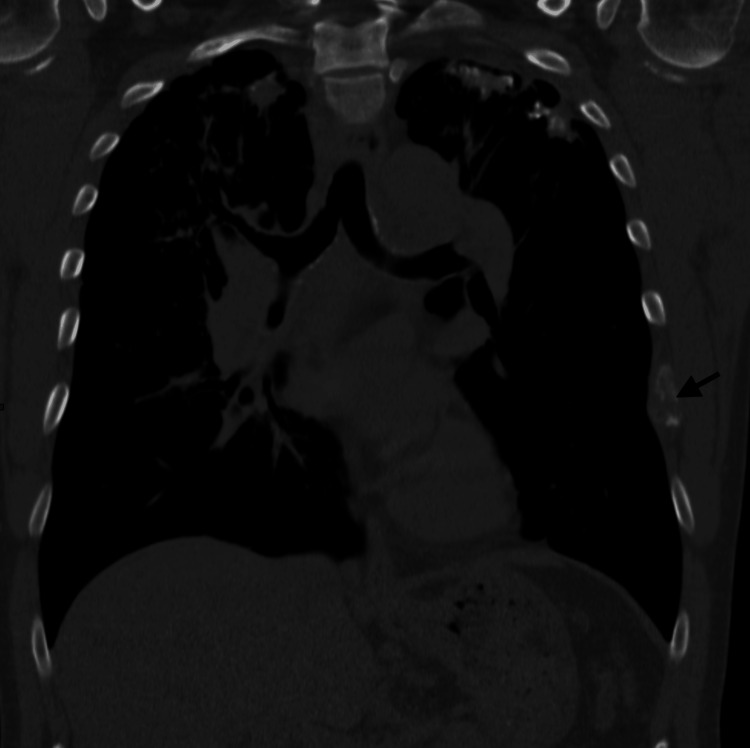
Coronal CT chest showing lytic lesion of the left lateral seventh rib (black arrow)
